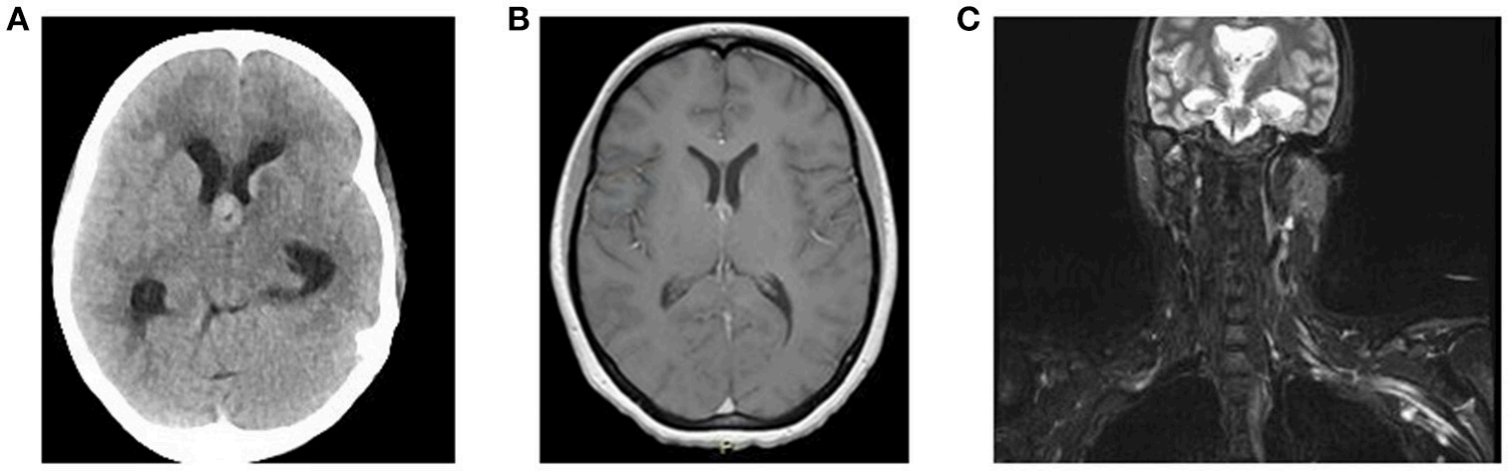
(A) Non-contrast CT Head demonstrates a lesion at the Foramen of Monro causing obstructive hydrocephalus. (B) MRI brain T1 axial study with contrast demonstrates enhancement of lesion at the Foramen of Monro. (C) MRI brachial plexus STIR sequence shows diffuse enlargement with marked T2 hyperintensity of the left brachial plexus.
